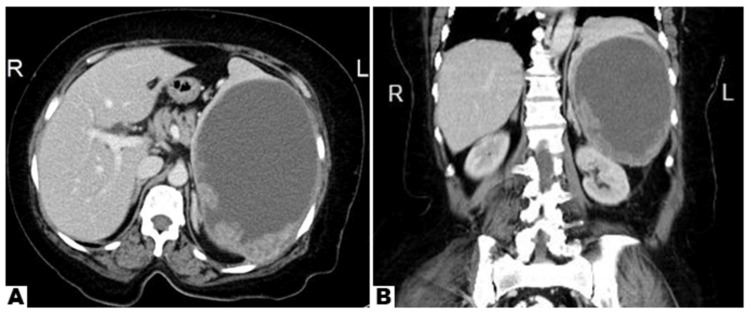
Contrast-enhanced CT scan of the abdomen (A) Axial and (B) coronal view showing a large unilocular thick-walled splenic cyst, consistent with splenic hydatid disease.
